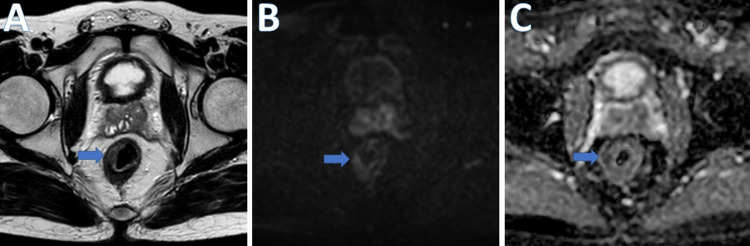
Post-CRT images A. T2W MR axial image. B. DWI. C. ADC image of a 35-year-old man with pathologically proven moderately differentiated adenocarcinoma of the mid- and lower rectum (T3N1M0) showing partial response with slight residual thickening and diffusion restriction at 9 O'clock position (blue arrows) CRT: chemoradiotherapy; DWI: diffusion-weighted imaging; ADC: apparent diffusion coefficient
